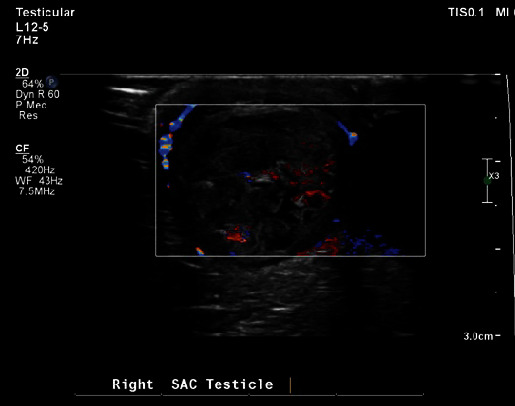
Coloured Doppler ultrasonography showing reduced vascularity in the right testis and increased size.
